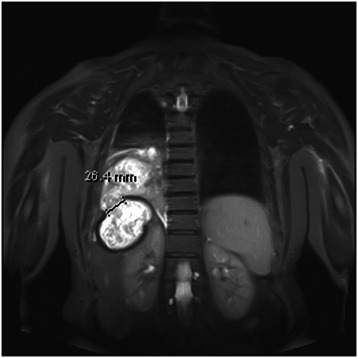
Magnetic resonance imaging scan demonstrating a 26.4 mm fistula in the background of hydatid liver disease. The fistulous communication is shown extending from the right lower lung lobe to the posterior aspect of right liver lobe
